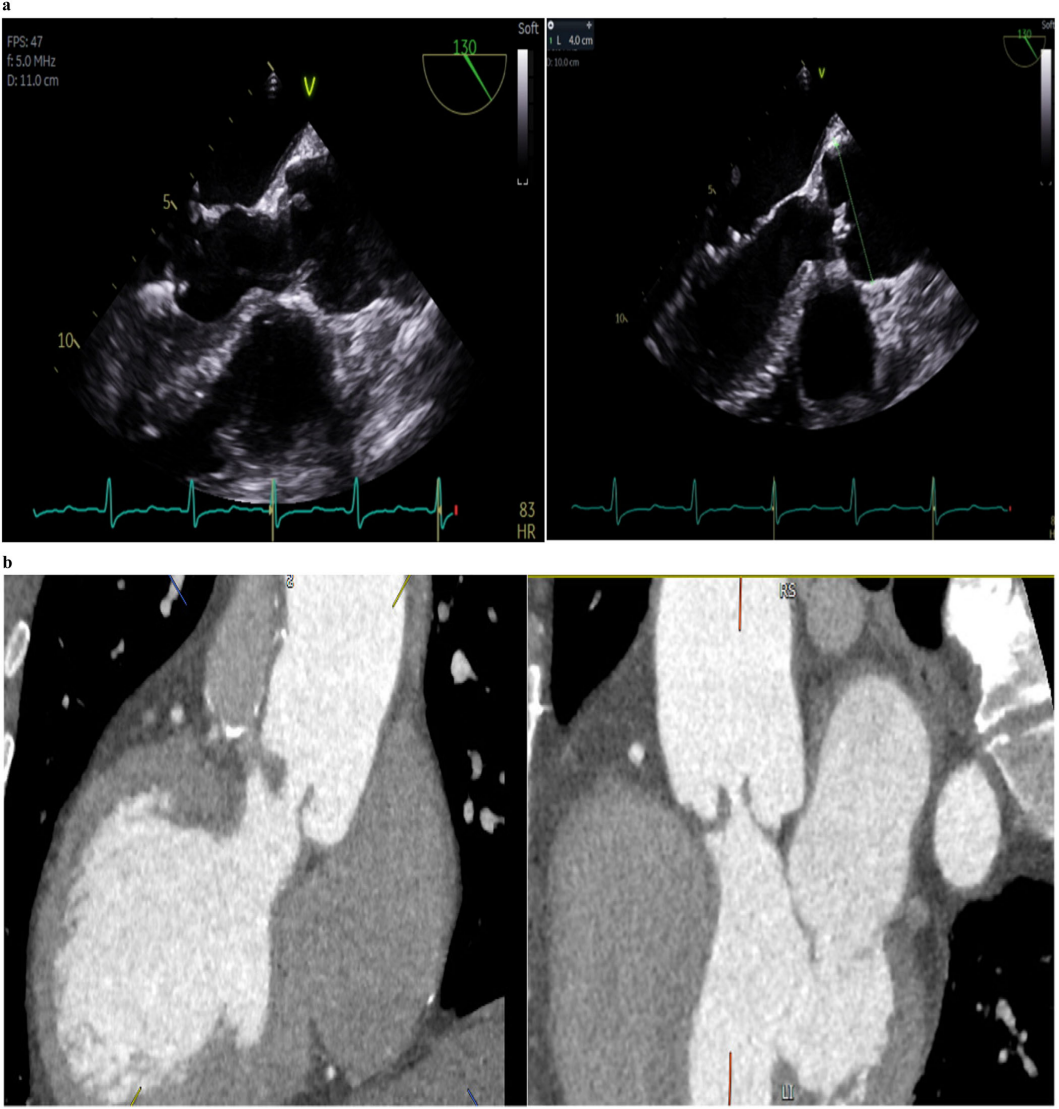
Pre‐procedural imaging. (a) TEE demonstrating the pathology. Severe neo‐aortic regurgitation due to fixed anterior leaflets and dilatation of neo‐aortic root causing central coaptation failure. (b) Pre‐procedural CT. Demonstration of the LVOT anatomy in the Ross patient with fixed anterior leaflets, and dilated neo‐aortic root causing central coaptation failure. The elongated LVOT formed by anastomosis between autograft and native LVOT forms the landing zone of the future TAVI valve. [Color figure can be viewed at wileyonlinelibrary.com]
